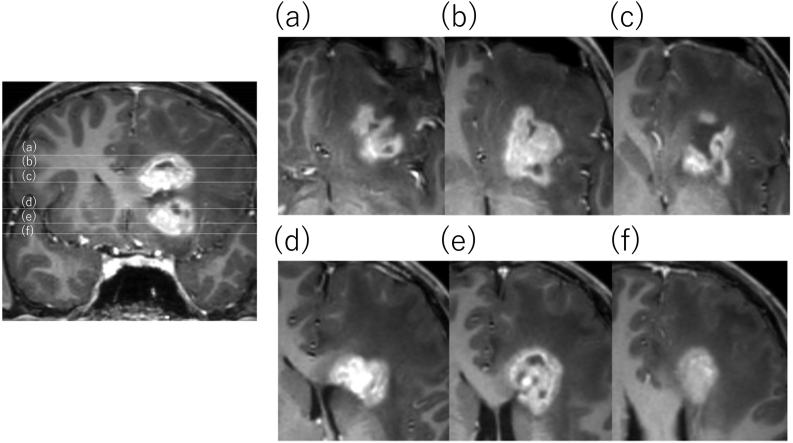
Gadolinium-enhanced magnetic resonance images. Two ring-enhancing lesions can be seen forming a unique shape that straddles the anterior horn of the left lateral ventricle: the inferior lesion extends from the anterior corpus callosum to the deep white matter, and the superior lesion extends from the basal ganglia to the orbital gyrus (each 3 cm in maximum diameter). A coronal section (left) and an axial section for each line drawn in the coronal section (right, a–f) are shown.
